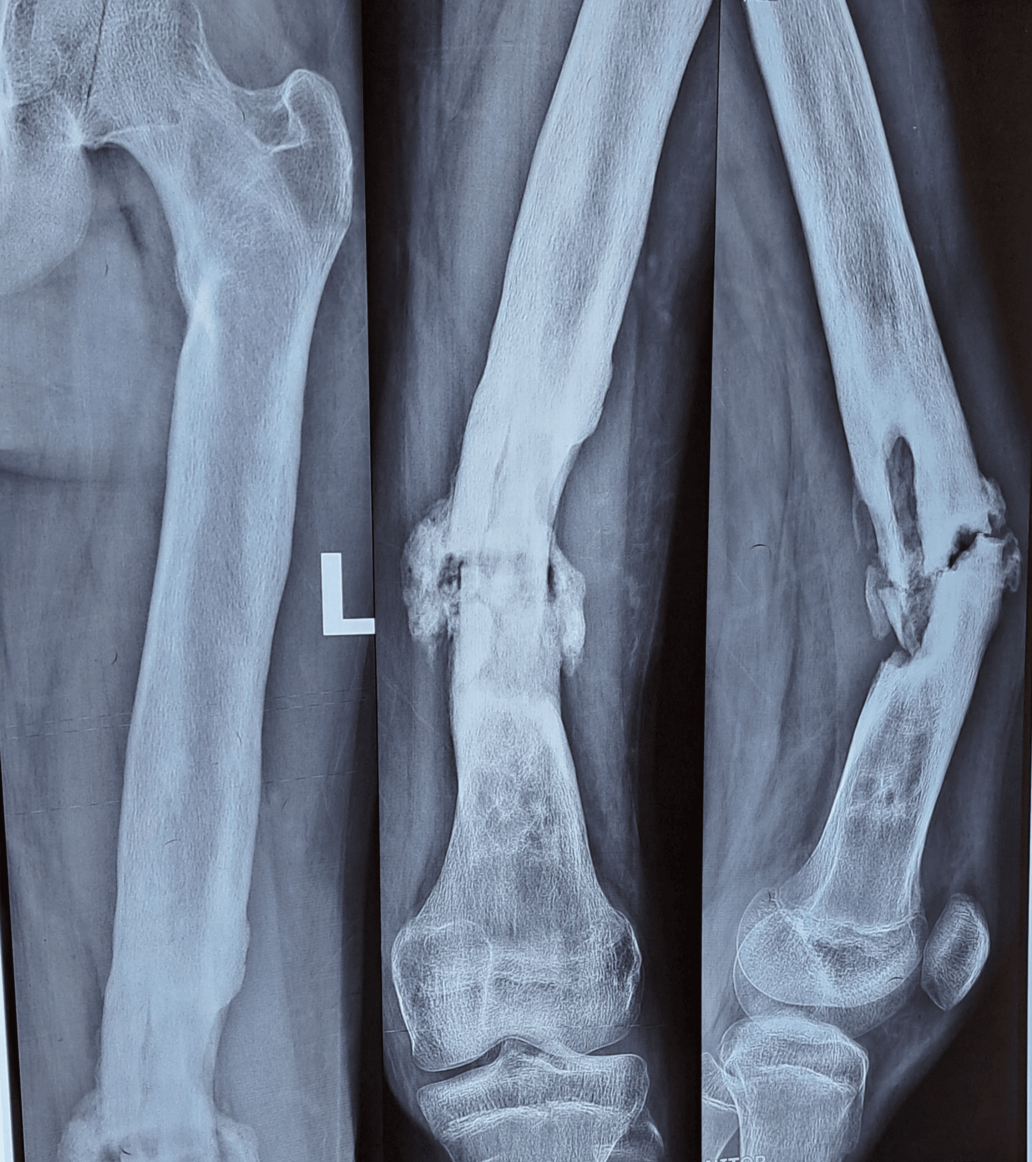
Preoperative X-ray of an infected nonunion of the femur with a bony defect
